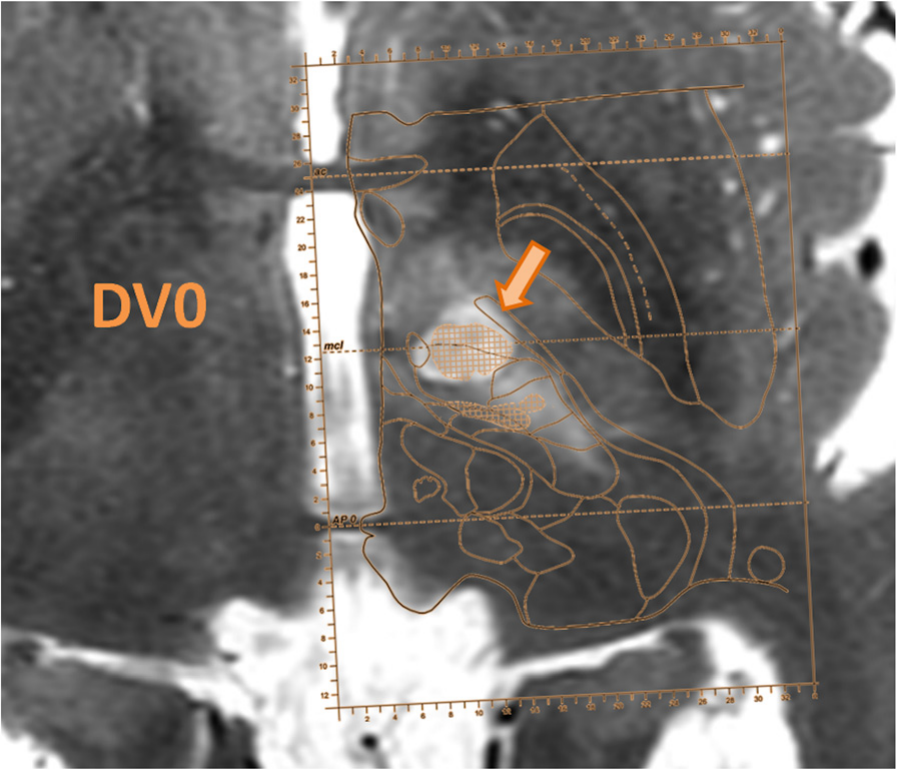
Superposition of atlas map over MR imaging. Axial MR T2-weighted (T2w) series taken at 2 days follow-up with superposition of the corresponding Morel atlas plane at the dorsoventral position 0 mm (DV0, the intercommissural plane). The hypodense lesion is exactly centered on the pallidothalamic tract as displayed on the atlas.
